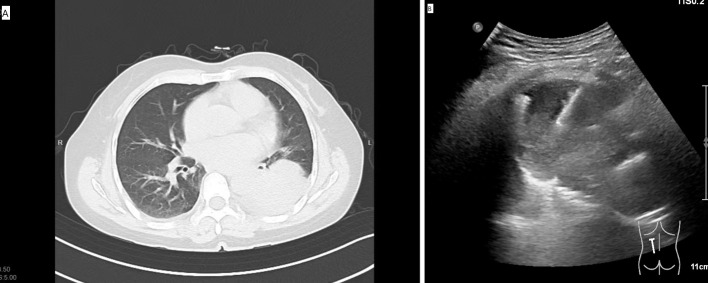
(A) The preoperative CT showed the SPL at left lower lobe. (B) The US-guided PNB procedure.
